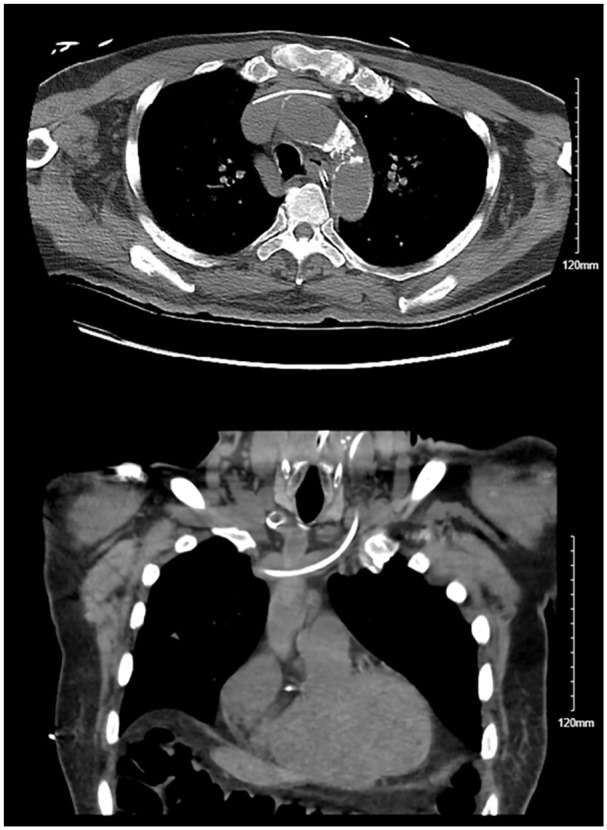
CT scan of the chest with axial (top) and coronal (bottom) images showing the catheter coursing into the Superior Vena Cava (SVC) and wedging into an anomalous right upper pulmonary vein that is draining into the SVC.
